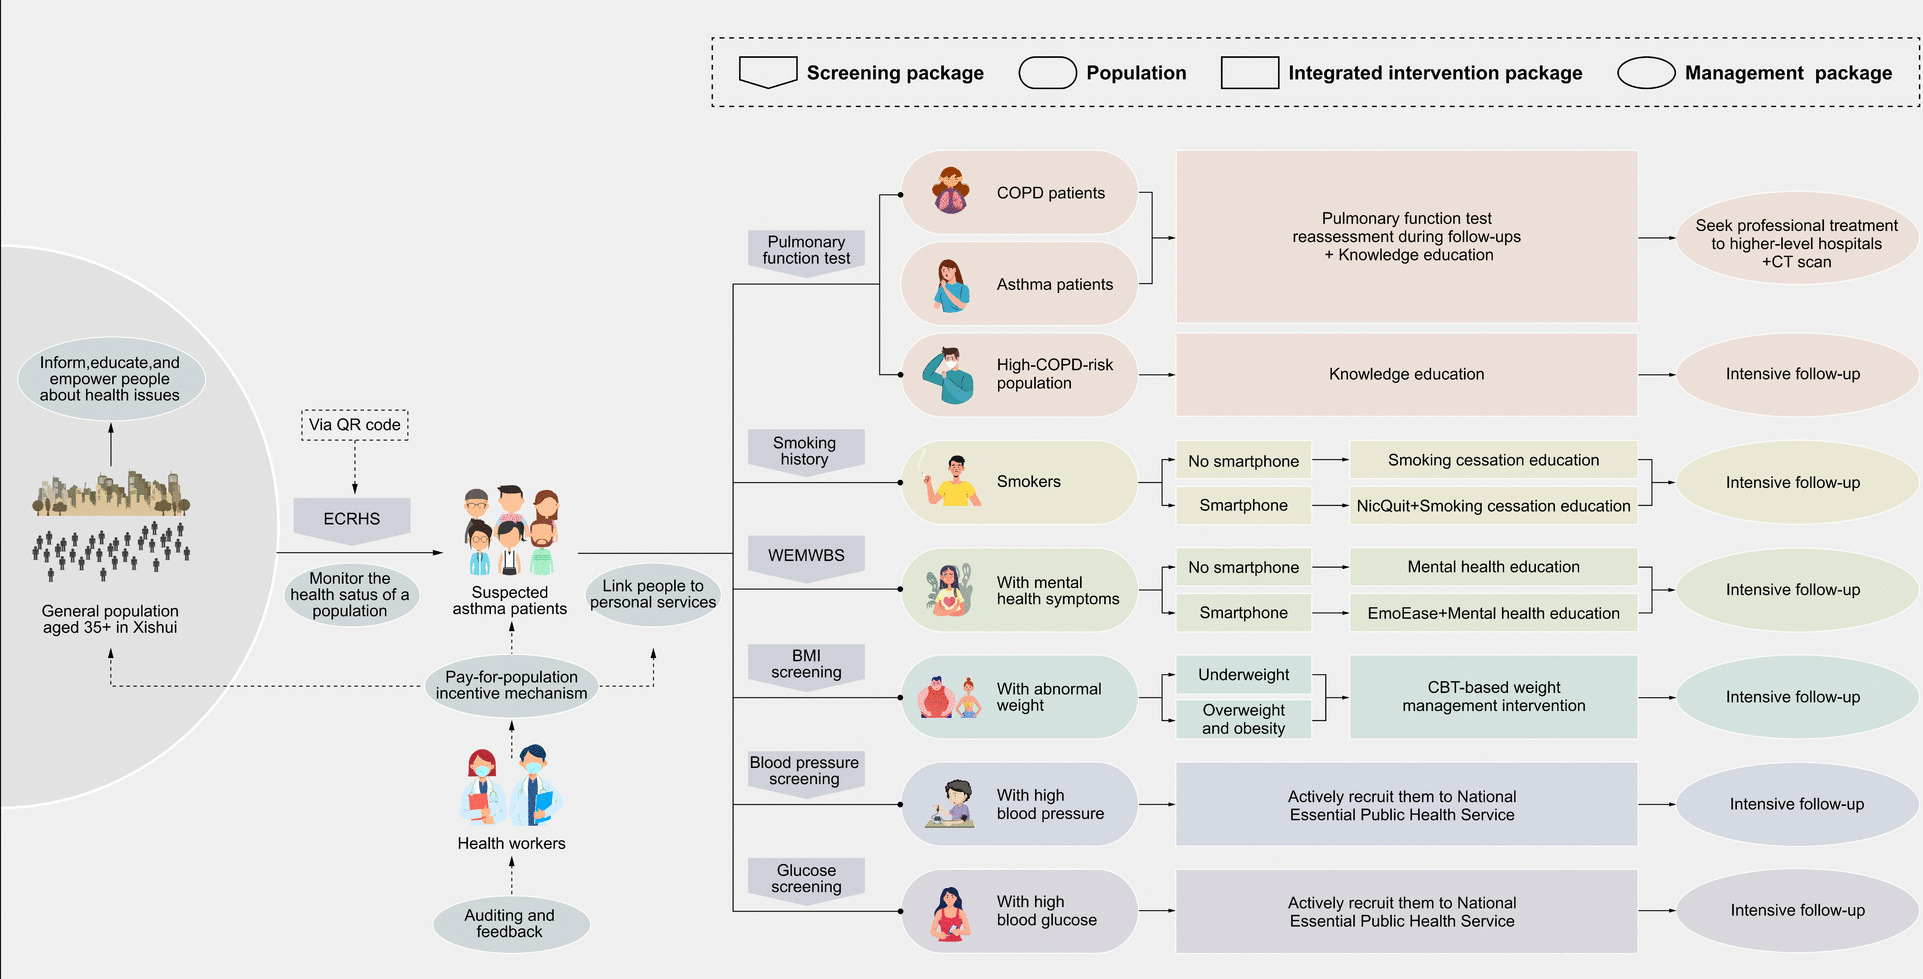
Integrated pathway of the multi-component intervention package. *Note: ECRHS* = *European Community Respiratory Health Survey; COPD* = *chronic obstructive pulmonary disease; WEMWBS* = *Warwick-Edinburgh Mental Well-being Scale; BMI* = *body mass index; CBT* = *cognitive behavioral therapy; CT* = *computed tomography; EmoEase* = *WeChat-based digital mental health intervention based on CBT; NicQuit* = *WeChat-based digital smoking cessation intervention based on CBT*
